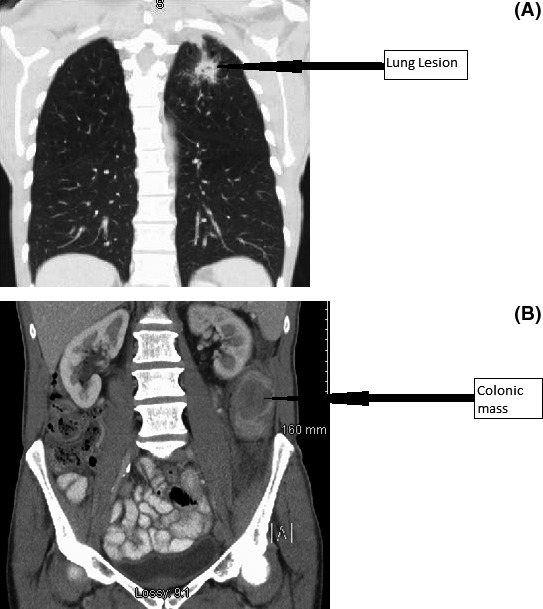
(A) Computed Tomography Thorax – lung lesion. (B) Computed Tomography Abdomen – abdominal lesion.
